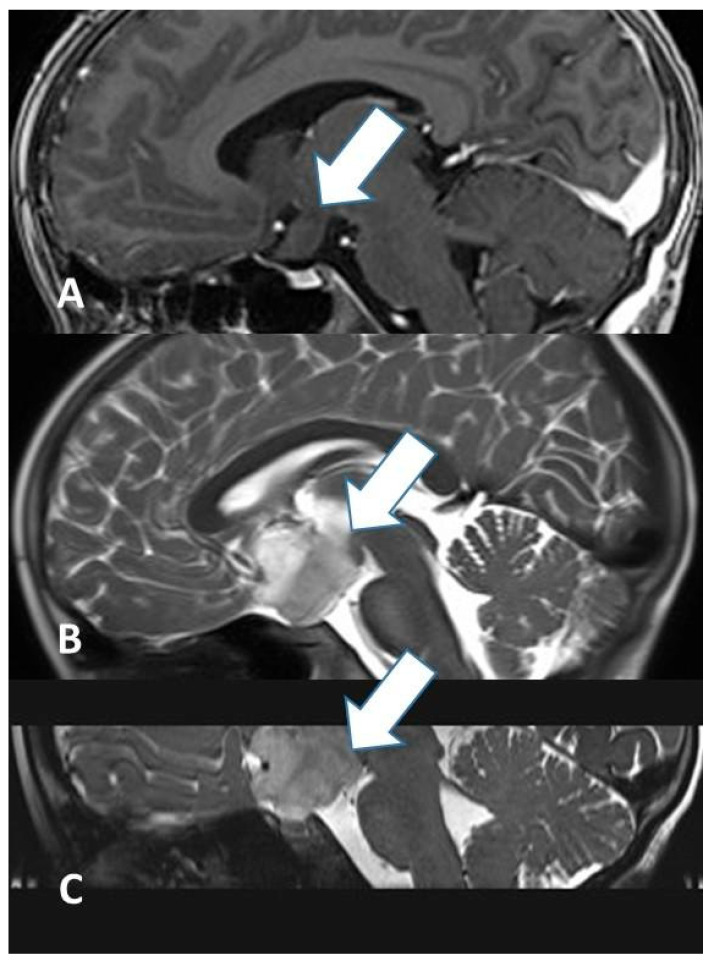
Longitudinal MRI surveillance of an optic pathway glioma in a patient with Neurofibromatosis type 1. Images (A–C) show the progressive growth of the optic glioma, indicated by the white arrows. A multidisciplinary strategy was implemented; to avoid permanent visual morbidity associated with radical resection, the patient underwent biopsy followed by chemotherapy.
